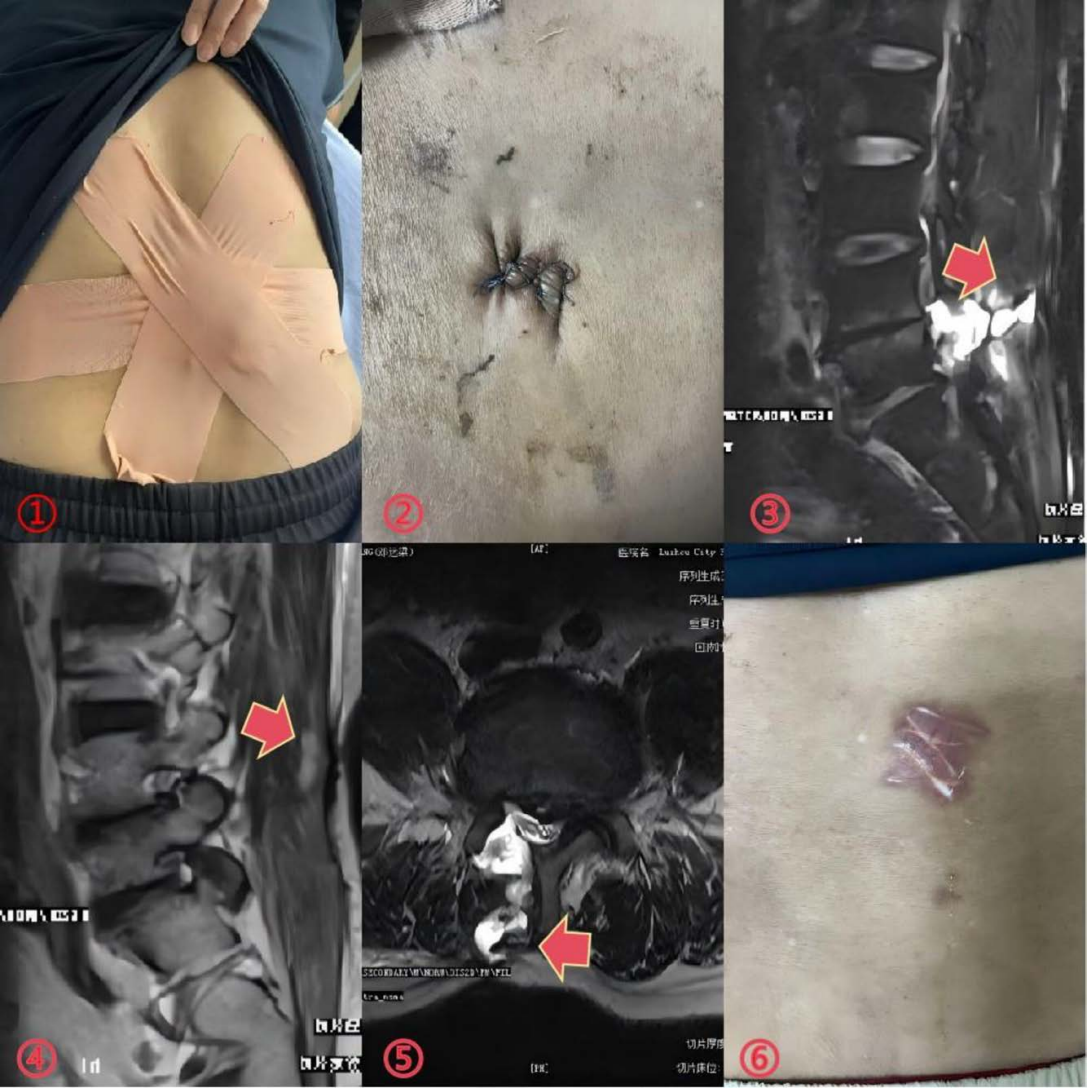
①: The lumbar mass was packed with gauze, and a pressure dressing was applied to manage the swelling and prevented further complications. ②: An attempt was made to close the subcutaneous sinus ostium through the skin, aiming to promote healing and prevented cerebrospinal fluid (CSF) leakage. ③④⑤: MRI revealed a reduction in subcutaneous effusion, indicating partial resolution of the fluid collection. However, the sinus remained patent. ⑥: CSF leakage was observed at the patient’s skin sutures.
